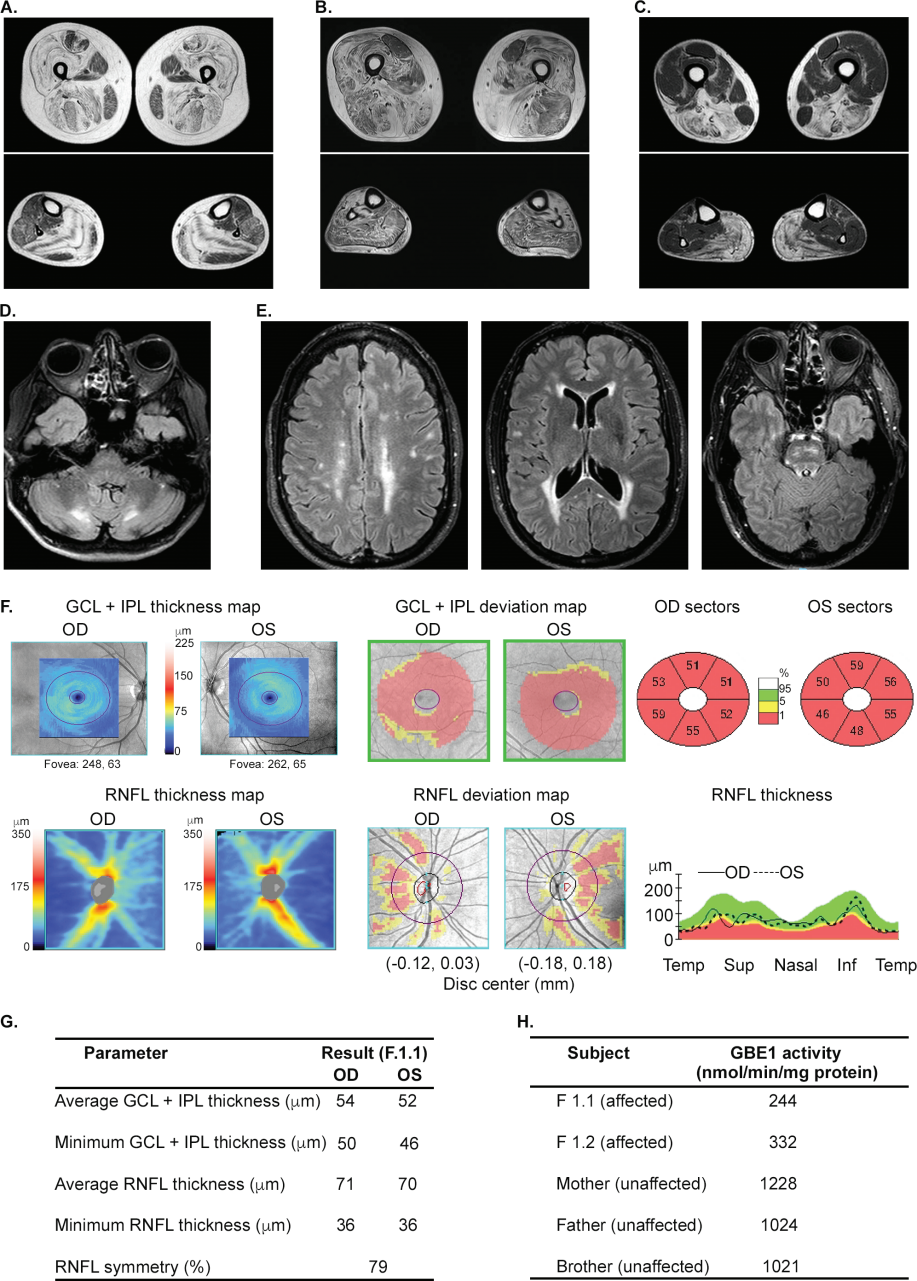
Examples of clinical test results aiding the genetic diagnosis by exome sequencing analysis. (A) T1-weighted axial images in F.3.1 patient with laminin α2 deficient LGMDR23 show peripheral fat replacement with central sparing in the vastus lateralis, soleus and gastrocnemius muscles, but the tibialis anterior is spared. The rectus femoris muscle shows increased signal around central fascia and periphery leaving a U-shaped less affected muscle. (B) The tibialis anterior is mostly replaced by fat, the quadriceps and adductor muscles are prominently affected, but the rectus femoris is spared in S.7 patient with Laing distal myopathy. In contrast with (A), multiple muscles show internal bands of fat infiltration. (C) Fat infiltration is seen in the adductor magnus, biceps femoris, semimembranosus, soleus and gastrocnemius muscles, followed by the semitendinosus, vastus and tibialis anterior muscles in S.2 patient with ADSSL1-related distal myopathy. (D) Typical symmetrical cerebellar white matter hyperintensities around dentate nucleus bilaterally in T2-FLAIR MRI in S.16 patient with cerebrotendinous xanthomatosis. optic neuropathy, leukoencephalopathy, and impaired cellular GBE1 activity in a family with adult polyglucosan body disease (APBD; E–G). (E) Axial T2-FLAIR images of brain show multiple discrete and confluent foci of high-signal in the subcortical white-matter, along the atria and occipital horns of the lateral ventricles, and along the white-matter tracts in the brainstem lesions. (F–G) Optical coherence tomography data showing bilateral atrophy of the macular ganglion cell-inner plexiform layer (GCL-IPL) and the retinal nerve fibre layer (RNFL), indicating neuronal death and axonal loss in optic nerves, respectively. Sup, superior; Inf, inferior. (H) The GBE1 enzyme activity measured in cultured skin fibroblasts of affected siblings and unaffected relatives.
